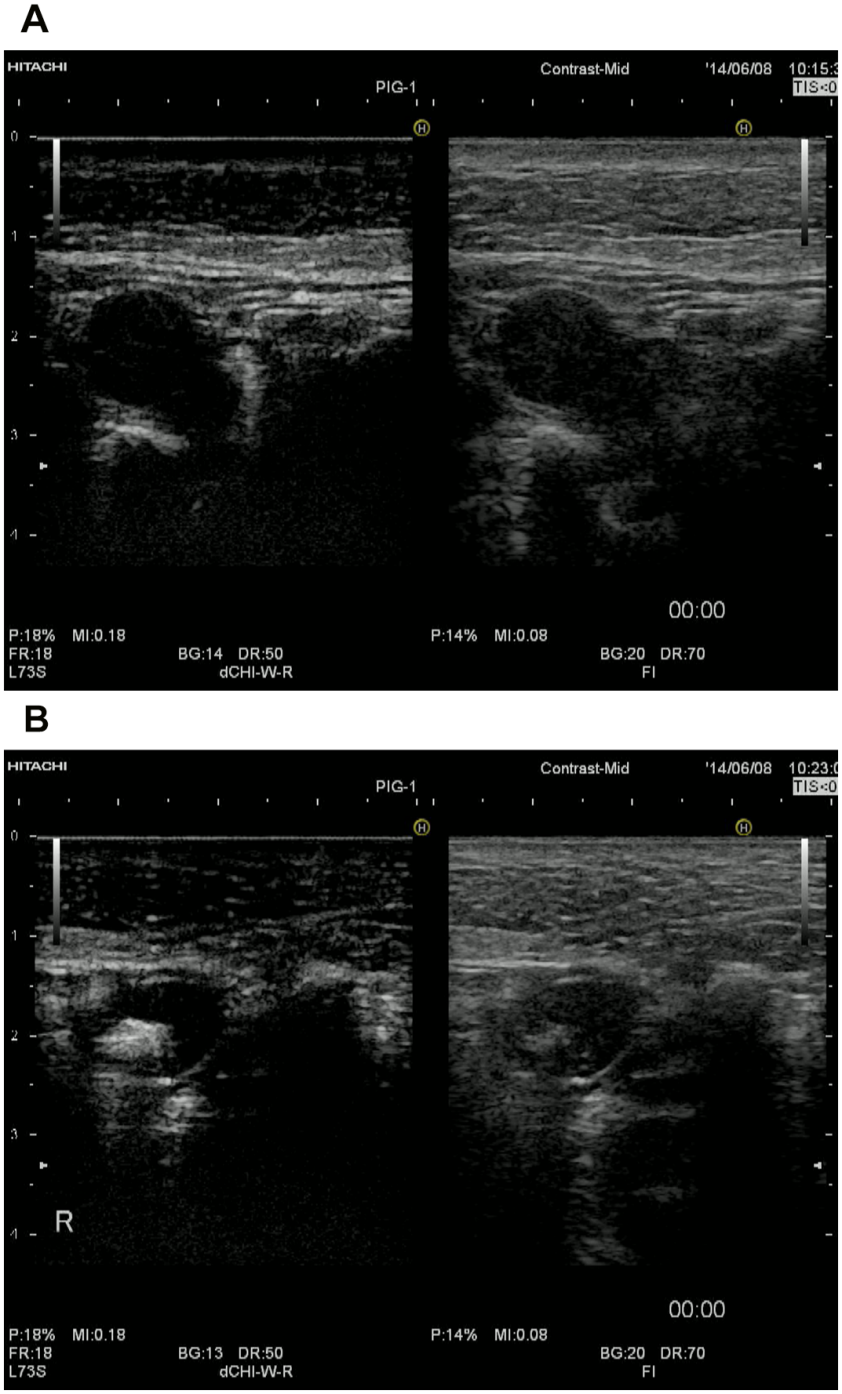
Sentinel node (SN) on the contrast-enhanced ultrasonography (CEUS) monitor. The left side of the monitor shows the contrast-enhanced mode and the right side shows the conventional B-mode view. a. View immediately after injection of the tracer mixture. The lymph node is not contrasted on the left side of the monitor. b. 20 minutes after injection of the tracer mixture, the lymph nodes were contrasted by Sonazoid. Note the high echogenicity of the SN due to the presence of contrast agent microbubbles on the left side. CEUS provided a clear image of the SN transcutaneously.
